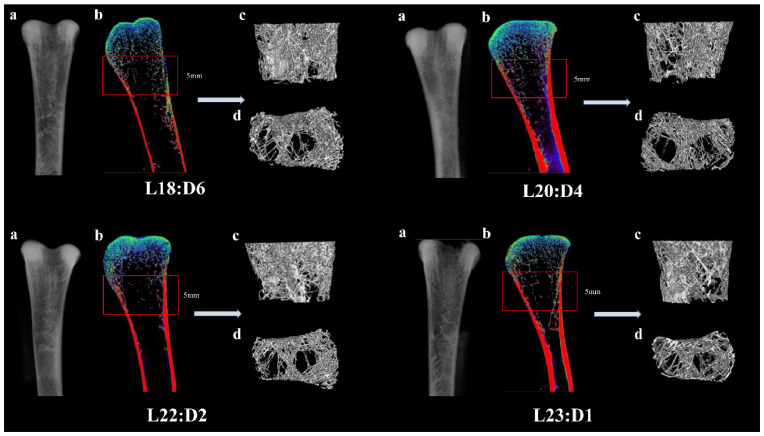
X-ray micro-computed tomography of the tibia structure in 63-day-old yellow-feathered broiler chickens. Figures (a–d) depict the three-dimensional surface view of the trabeculae, the coloured two-dimensional microstructure, the horizontal view of the trabecular microstructure in the selected region, and the bird’s-eye view of the trabecular microstructure within the selected region, respectively. 18L:6D: 18 h of light and 6 h of dark; 20L:4D: 20 h of light and 4 h of dark; 22L:2D: 22 h of light and 2 h of dark; 23L:1D: 23 h of light and 1 h of dark.
